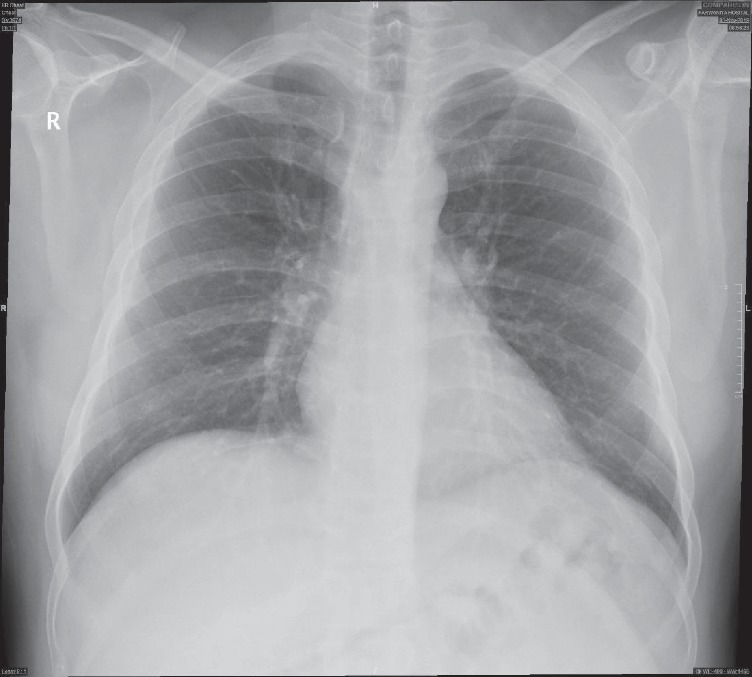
Follow-up chest radiograph showing no evidence of recurrence of pneumothorax.
